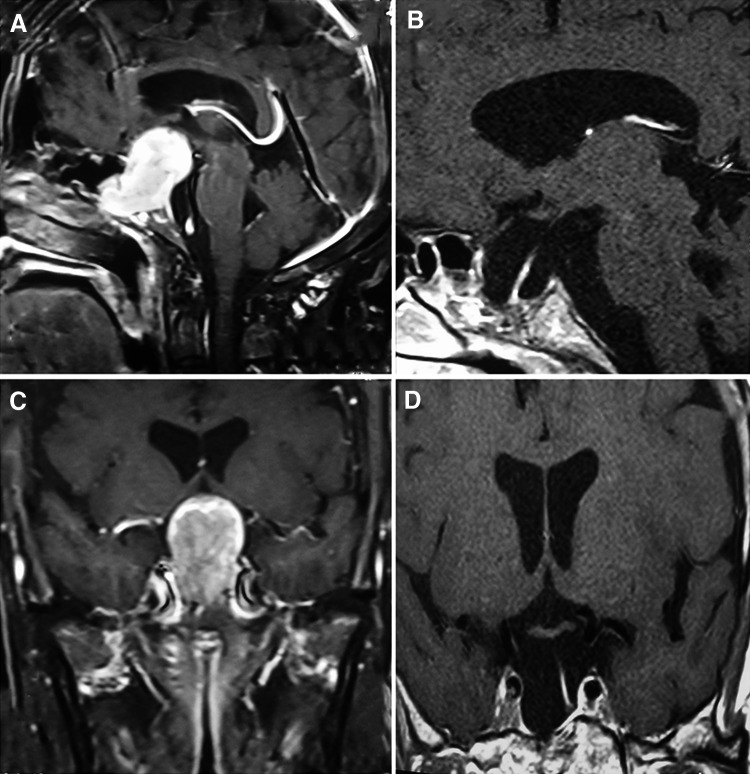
MRI images of one nonfunctioning adenoma. A, C Before surgery, B, D gross total resection after surgery
